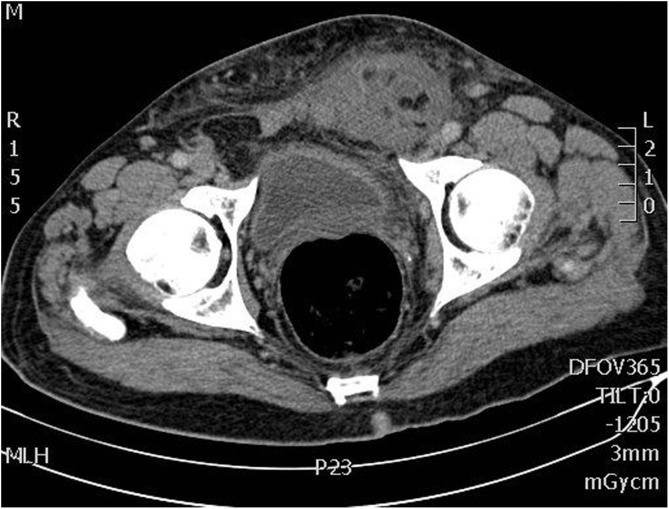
CT Scan revealing a left incarcerated inguinal hernia.
